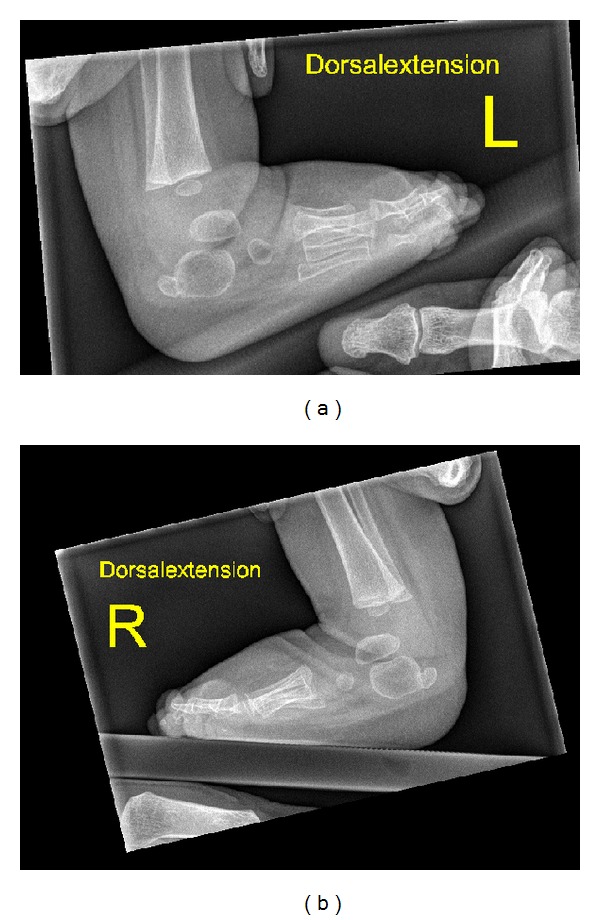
(Patient 2) Lateral feet radiograph showed the juxtacalcaneal ossific centres.
